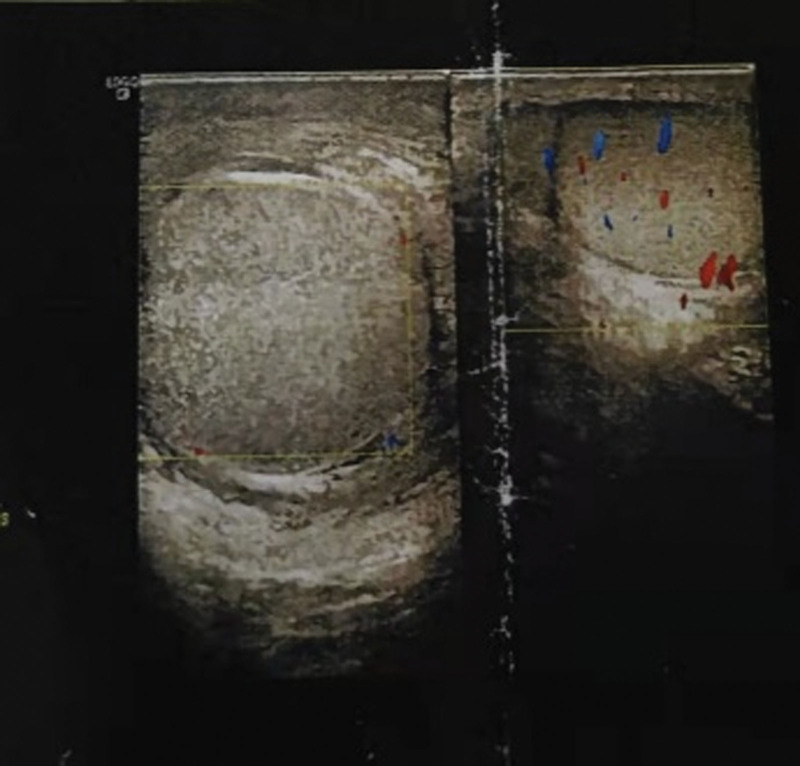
Doppler ultrasonography reveals right epididymitis, right scrotal wall swelling, and right testicular torsion.
